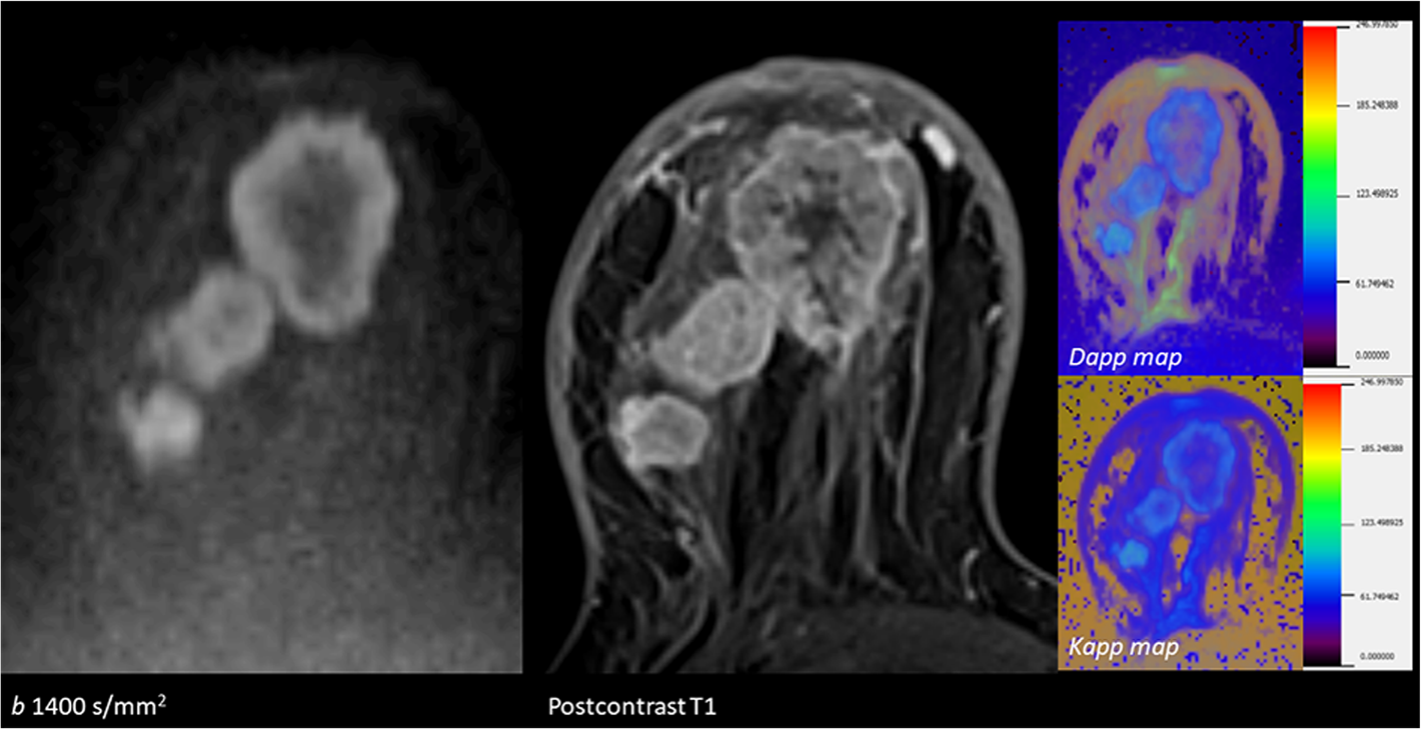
Diffusion kurtosis imaging analysis of multicentric invasive breast carcinoma. DW-MRI, postcontrast T1, and corresponding D apparent (Dapp) and K apparent (Kapp) maps
